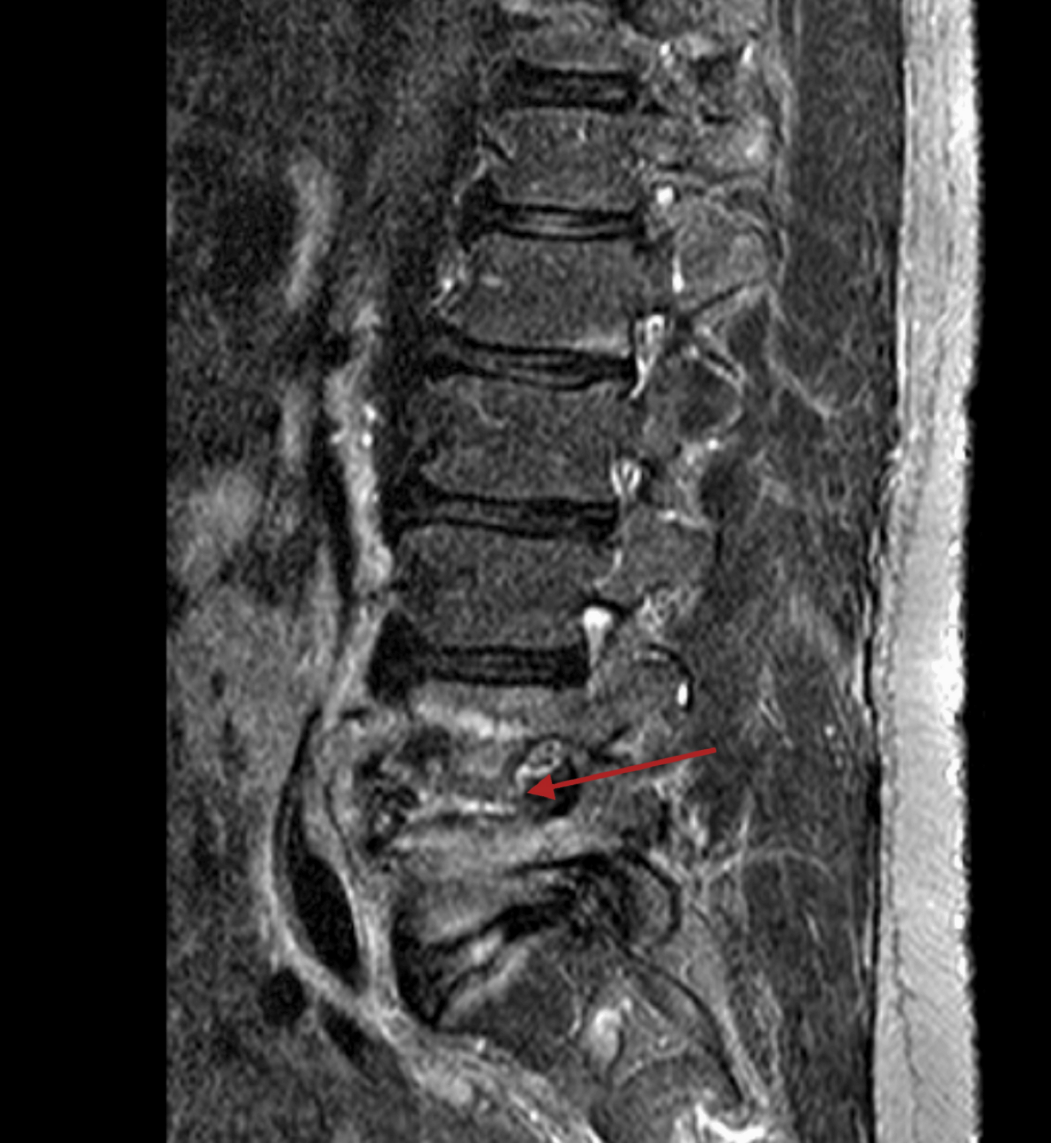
MRI lumbar spine with and without contrast (SAG STIR series) demonstrating osteomyelitis at L4-L5, with potential marrow edema (red arrow). Disc protrusion is also seen at L5-S1. There is central stenosis at L3-L4 (mild to moderate), L4-L5 (moderate to marked), and L5-S1 (moderate).
